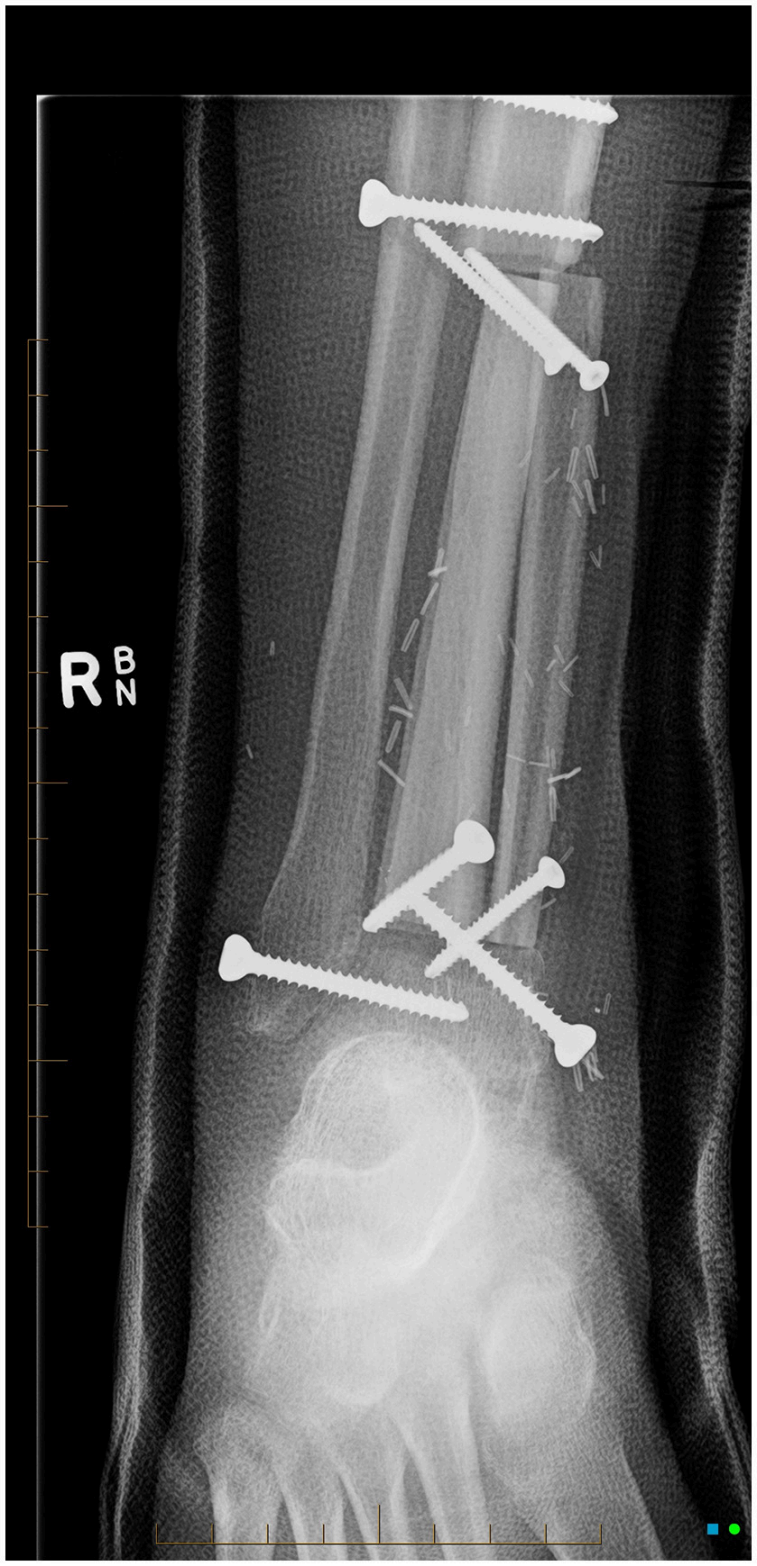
AP x-ray of right lower leg, cannulated screws transfix the fibular bone graft to the distal right tibial diaphysis.
